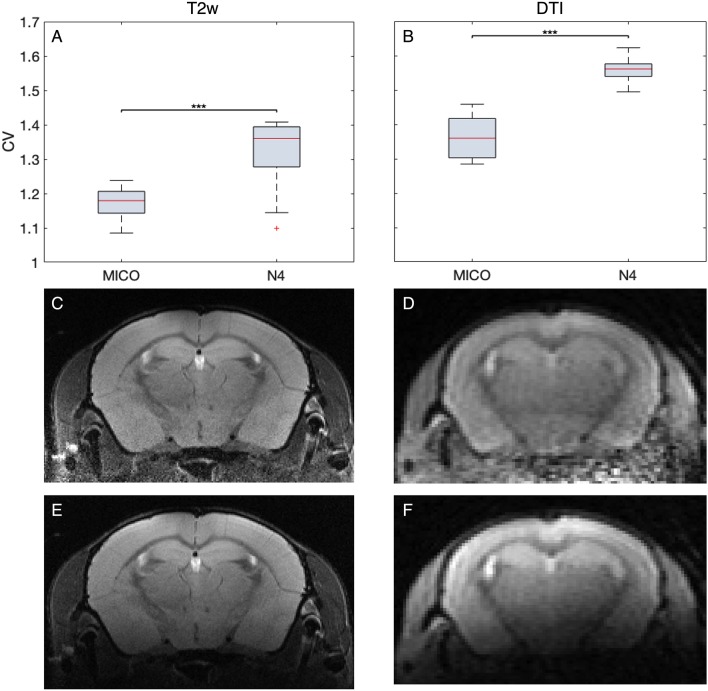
Quantitative and qualitative comparison of MICO and N4 bias-field correction. The calculation of the degree of homogeneity revealed lower coefficient of variations (CV) for MICO compared to N4 for 22 T2w and 10 DTI measurements (A,B). Representative MR images comparing MICO (C,D) and N4 (E,F) bias-field corrected images for T2w and DTI, respectively.
